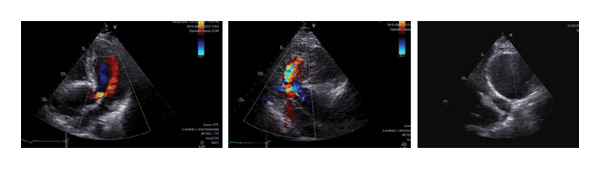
Transthoracic echocardiogram showing aortic regurgitation and aortic dilatation.
